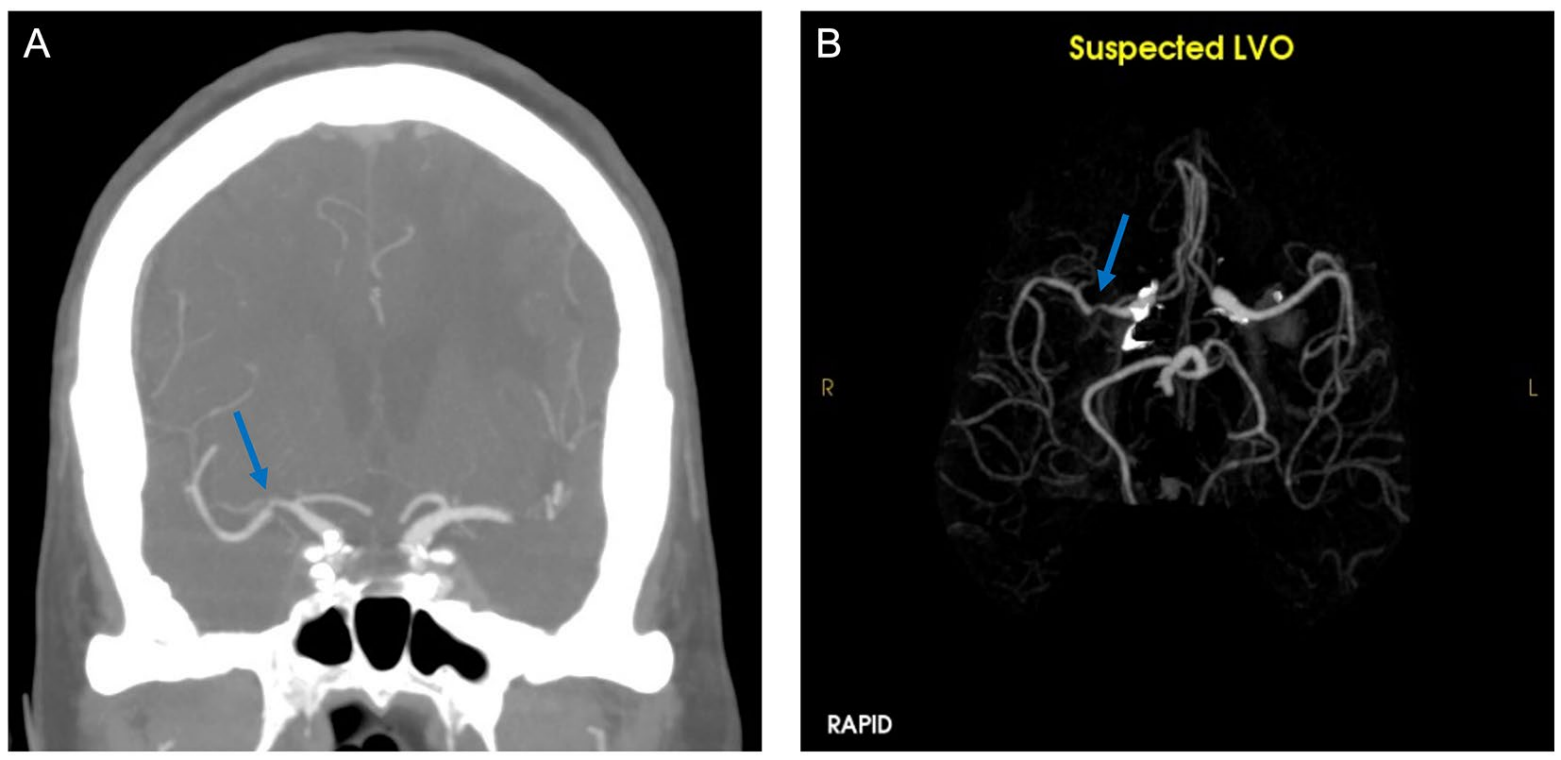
CTA (A) demonstrates stenosis of the right middle cerebral artery. RAPID LVO output (B) showed a suspected LVO (blue arrow).
